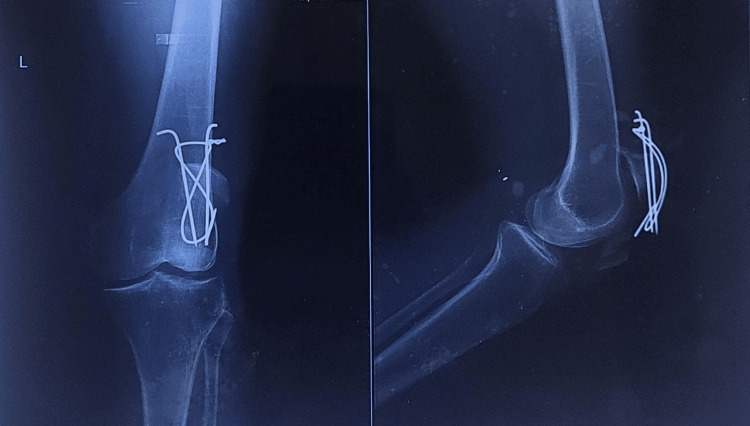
Plain radiograph obtained after first tension band wiring surgery of fractured patella. Post-operative anteroposterior and lateral knee radiographs showing patella fracture reduction and internal fixation with tension band wiring. Note the distracted fragments indicating loss of fracture reduction
